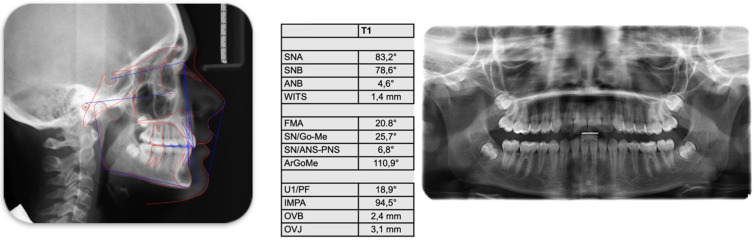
Final panoramic x-ray and final teleradiography and cephalometric analysis.
